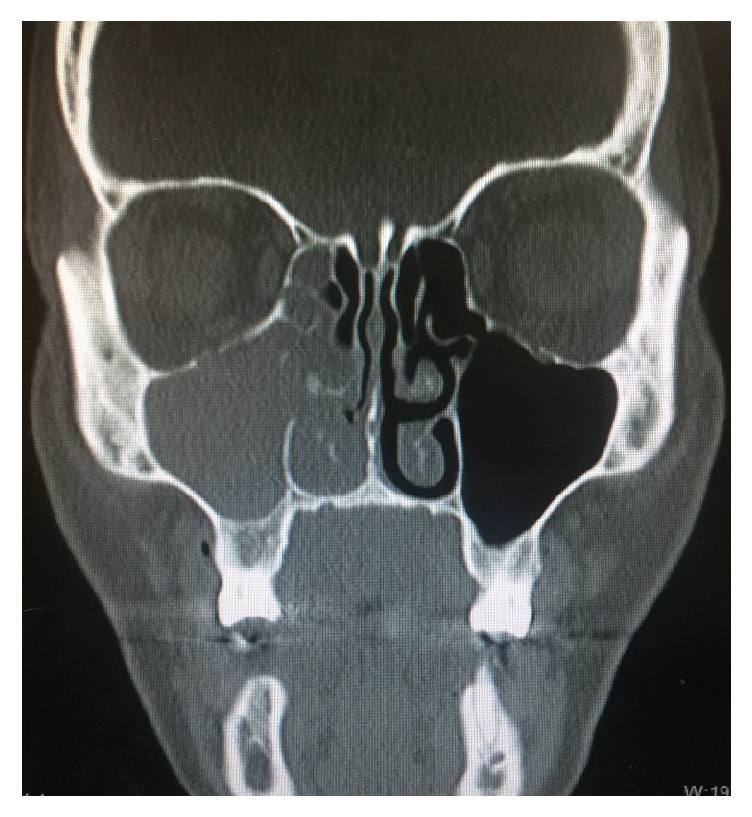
A coronal CT image demonstrating complete opacification of the right maxillary sinus with soft tissue extending through the ostiomeatal complex and into the right nasal cavity.
